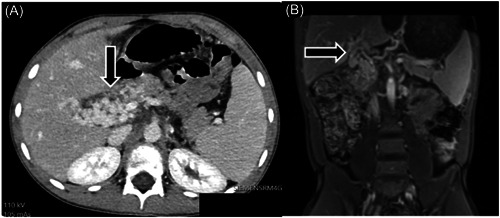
(A) Axial CT image of extensive varices at the porta hepatis. (B) Coronal T1 fat‐saturated postcontrast MRI showing massive varices at the porta hepatis.
